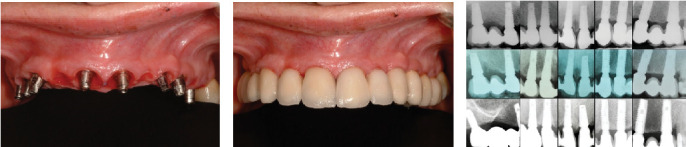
(a) Soft tissue sculpted after 12 weeks. (b) Definitive cement-retained fixed porcelain fused to metal partial dentures with sculpted soft tissue at day of cementation. (c) Periapical radiographs at delivery time (top), 10-year (center) and 18-year (bottom) follow-up findings showing maintenance of bone at level of implants and interproximal bone without modifications.
